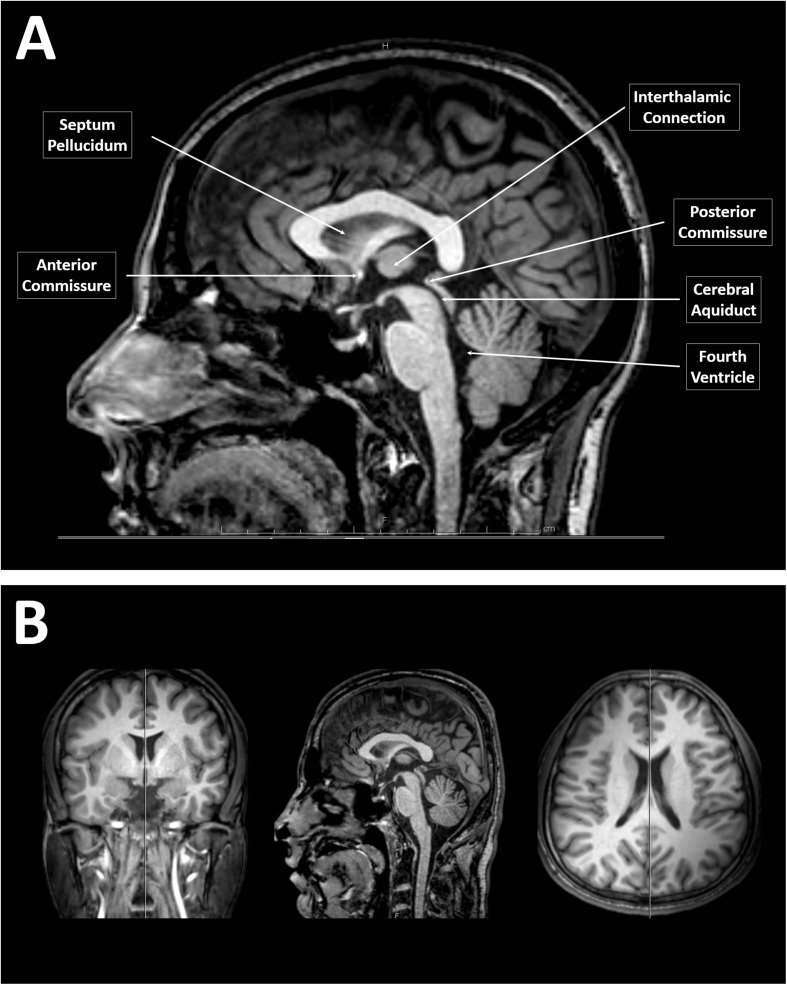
Identification of a midsagittal section from magnetic resonance (MR) images of the brain. (A) A midsagittal MR image of the brain showing the clear separation between the tectum and tegmentum of the midbrain by the cerebral aqueduct, a visible fourth ventricle and cerebellar vermis, clear distinction of the septum pellucidum and interthalamic connection, and visible anterior to posterior commissures. (B) T1-weighted MR images of the brain of a younger adult male that simultaneously showing the coronal, sagittal, and horizontal series of the sections provided by the Picture Archiving and Communication System software.
